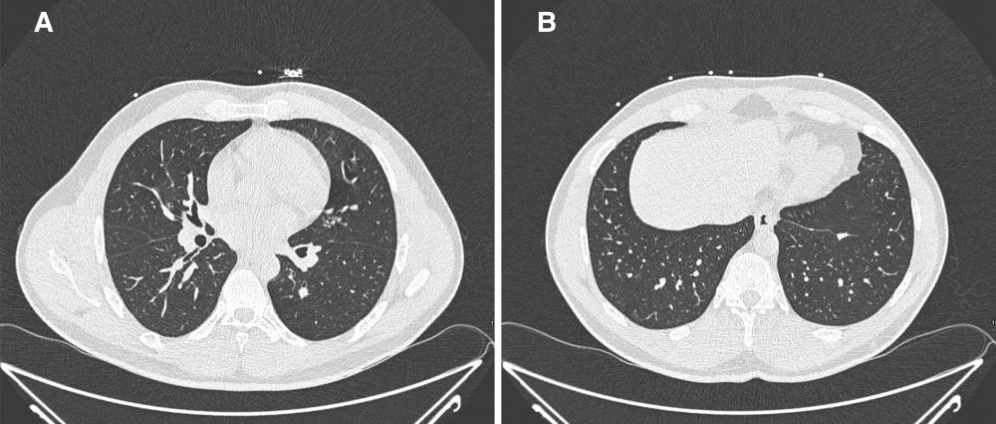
Non-contrast high-resolution computed tomography of the thorax displaying multiple prominent mediastinal and bi-hilar lymph nodes measuring up to 11 mm in short axis diameter (*A*) and multiple subcentimetre pulmonary, subpleural, and perifissural nodules (*B*).
